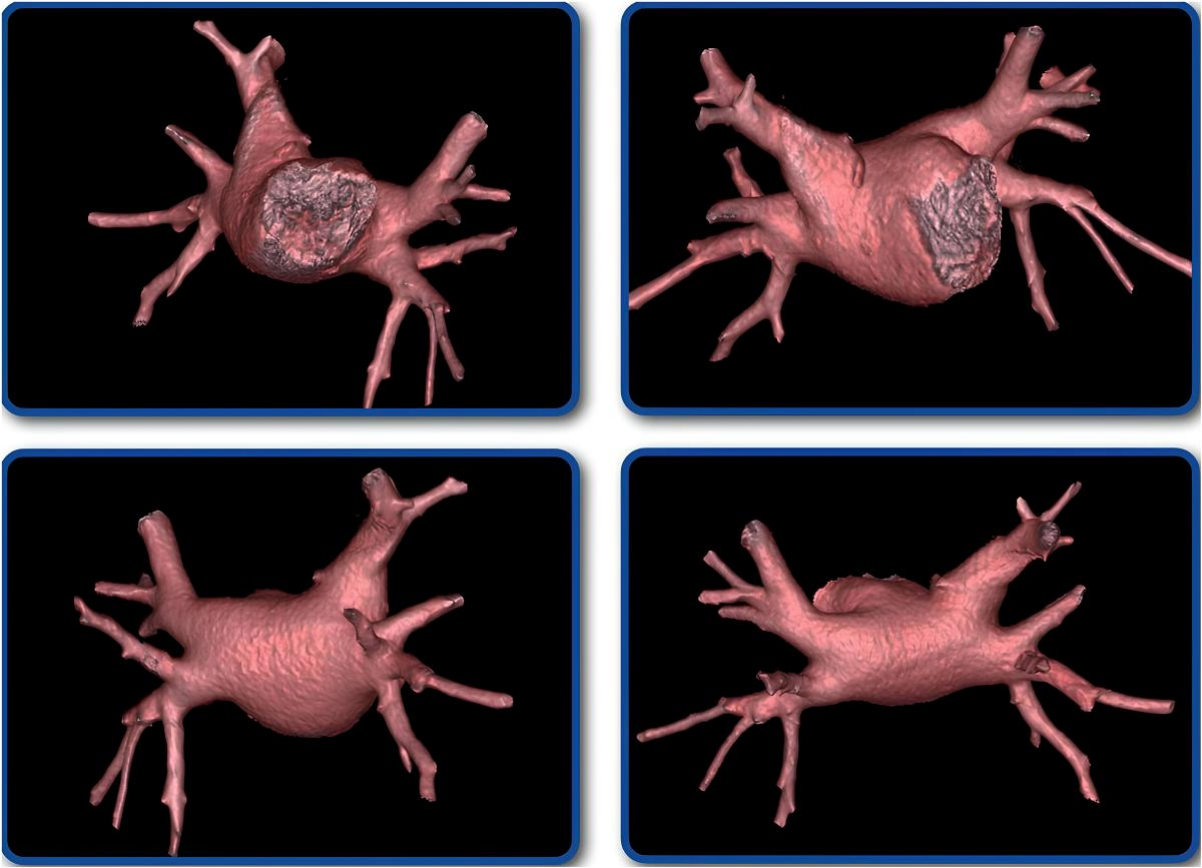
Angio-computed tomography of the left atrium demonstrating the absence of the left atrial appendage.
